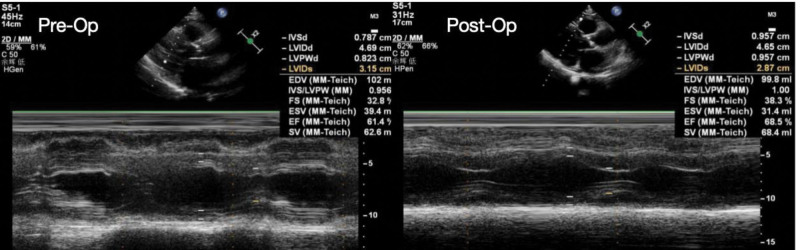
A comparison of pre- and post-operative echocardiograms demonstrated significant improvements in both cardiac structure and function: the left atrial diameter (LAD) decreased from 44.4 mm to 42.6 mm, the left ventricular end-diastolic diameter (LVIDd) reduced from 46.9 mm to 46.5 mm, while the ejection fraction (EF) increased from 61% to 69%, indicating markedly enhanced left ventricular systolic function and suggesting favorable therapeutic outcomes from the surgical intervention.
